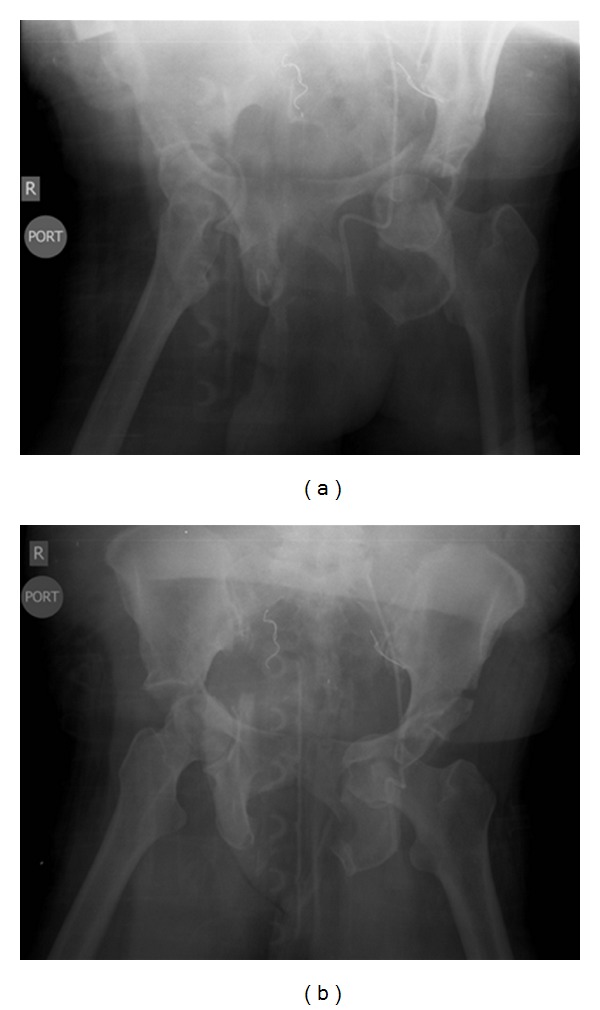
(a) Anterior-posterior radiographic image of pelvis—courtesy of the Botsford General Hospital Radiology Department. (b) Anterior-posterior radiographic image of pelvis—courtesy of the Botsford General Hospital Radiology Department.
